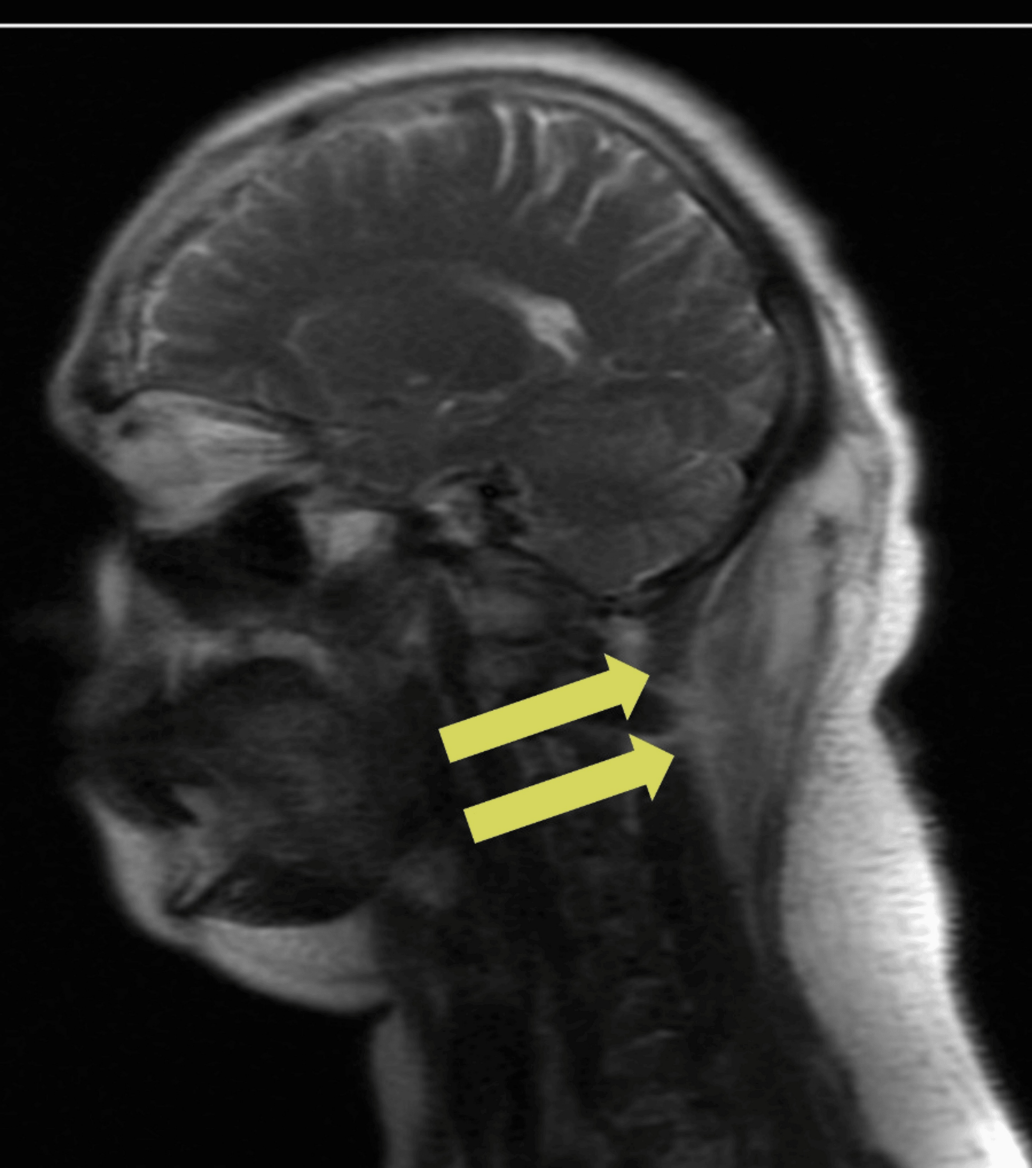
MRI showing abscess within the semispinalis capitis muscle and bone changes suggestive of osteomyelitis Axial T1-weighted post-contrast MRI of the upper cervical spine performed on hospital day 1 shows a 4 × 2 × 6 cm abscess within the right semispinalis capitis muscle. Surrounding inflammatory changes are present, suggestive of underlying osteomyelitis. There is no involvement of the spinal canal or intracranial structures.
